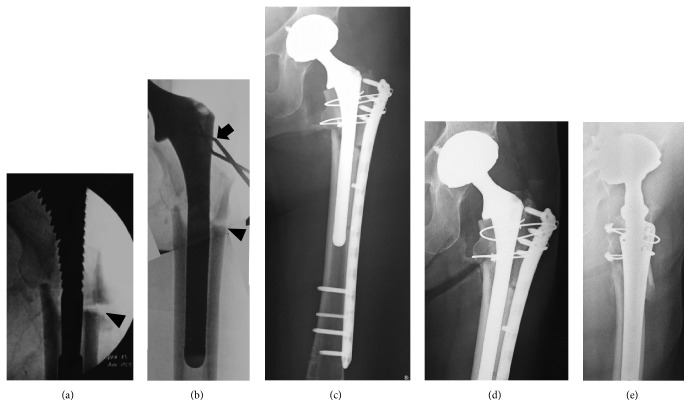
(a) A trial broach with the optimum size was inserted, but reduction was not enough (arrowhead). (b) Varus of the proximal bone fragment was prevented using the Blocking Pin Technique (arrow), and the stem was placed in the optimal position (arrowhead). (c) The osteosynthesis-combined one-staged total hip arthroplasty. (d) AP and lateral views on radiograph obtained after second surgery six months show complete union of the fracture.
